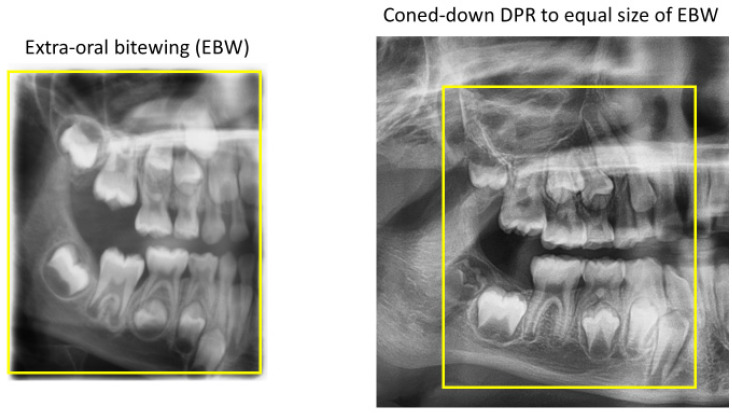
The extra-oral bitewing (EBW) of the posterior deciduous (baby) teeth produced by an EBW program on a DPR unit. The overlap between the adjacent proximal surfaces of the posterior teeth is either absent or minimal compared with the more extensive overlaps between them on the DPR. Additionally, the EBW covers more of the dentoalveolus, exhibiting both erupted and unerupted teeth and their root development. The extent of the EBW relative to the DPR is denoted by the yellow box. These last features are more important to the dentist who cannot otherwise evaluate the primary or mixed dentition of children either clinically or by the smaller, traditional intra-oral bitewings.
